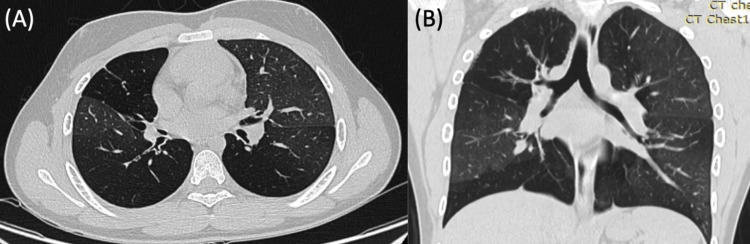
(A) Axial HRCT of the chest shows bilateral mosaic attenuation pattern approximately ten months after symptom onset; (B) Coronal HRCT with stable pulmonary disease post-FAM treatment approximately one year later showing similar mosaic attenuation pattern, without improvement HRCT: High-resolution CT; FAM: Fluticasone oral inhalation, azithromycin, montelukast
